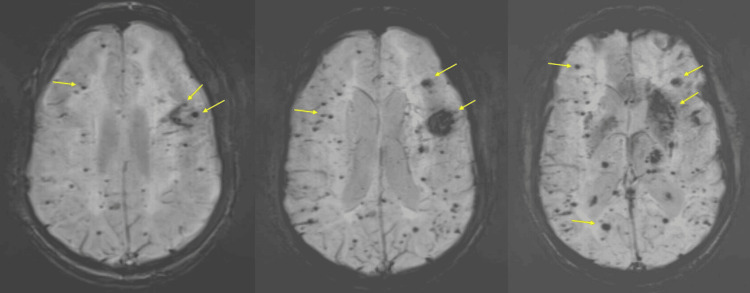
MRI Brain Without Contrast SWI Sequence The image provides a series of axial MRI brain SWI images. Indicated by the yellow arrows are susceptibility hypointensities throughout the bilateral cerebrum representing microhemorrhages. In the panel, there is a prominent juxtacortical area of susceptibility in the left frontal lobe as well as in the left basal ganglia as shown by the yellow arrows. MRI: magnetic resonance imaging, SWI: susceptibility-weighted imaging.
